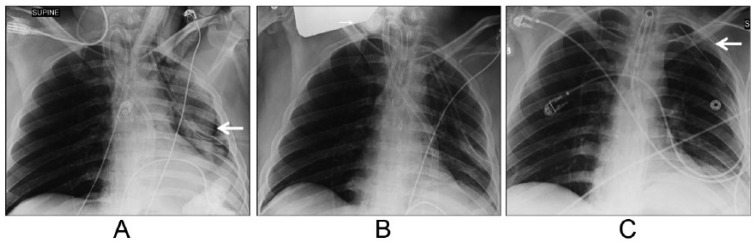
Results of patient’s X-ray examinations: (A) intraoperative chest X-ray demonstrating left pneumothorax; (B) intraoperative chest X-ray after left chest tube placement; (C) postoperative chest X-ray.
